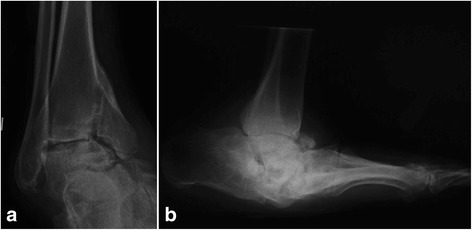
The radiographic characters of DNOAP. The fracture type (a) and the dislocation type (b)
